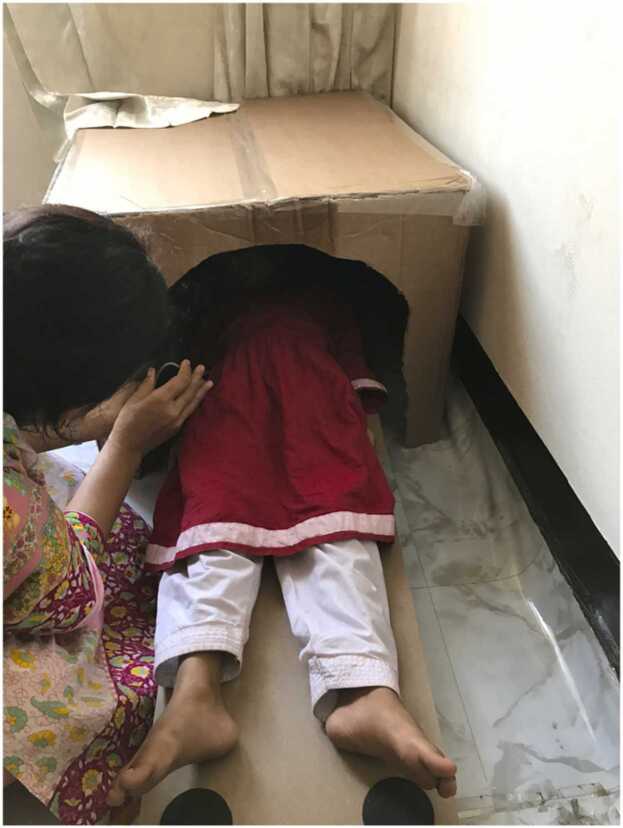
Cardboard mock scanner at MRI facility.
